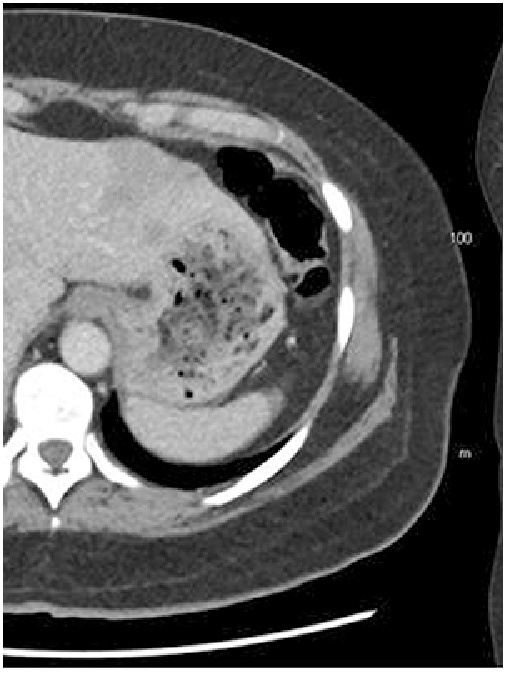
Follow-up CT scan 6 months later demonstrating new liver metastases (axial and coronal views).
